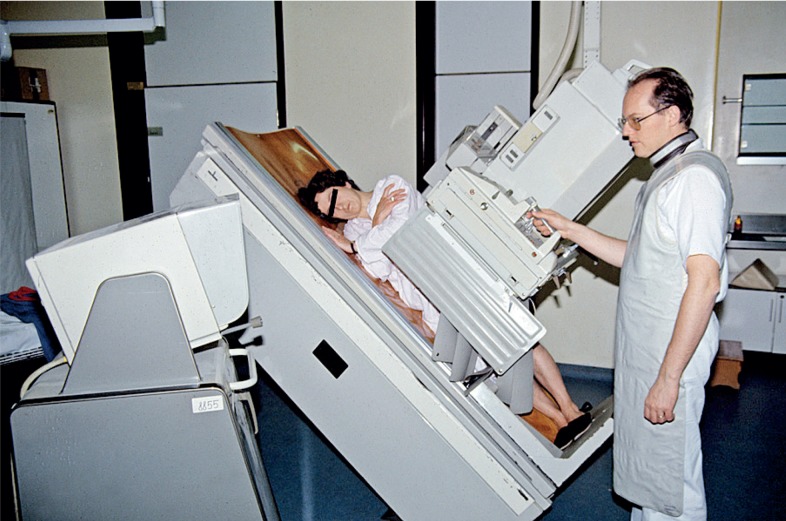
Siemens Sireskop (1974–1980s). With electronic image intensification, examination could be made in normal light and recorded on x-ray film or videotape. Maks Kadivec is performing a neuro-radiological procedure. Similar Siemens unit (next door) modified with rapid-change film holder (portable puck) allowed multiple images essential for studies of the cardiovascular system (1964 – early 1990s). There was as well modified homemade cassette changer (“boben”) for peripheral angiography.
